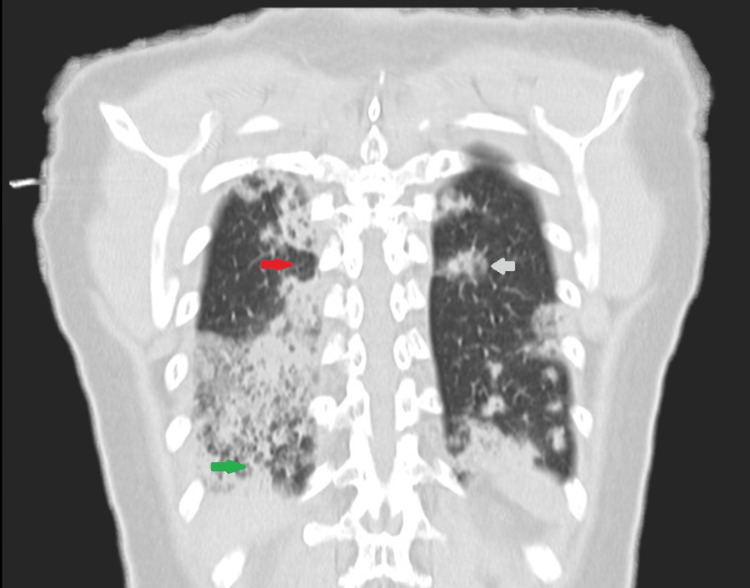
Thoracic CT-scan Red arrow shows enfisema bubbles; green arrow shows a mosaic pattern; blue arrow shows a nodular formation with soft-tissue density (impacted mucous vs adenomegaly)
